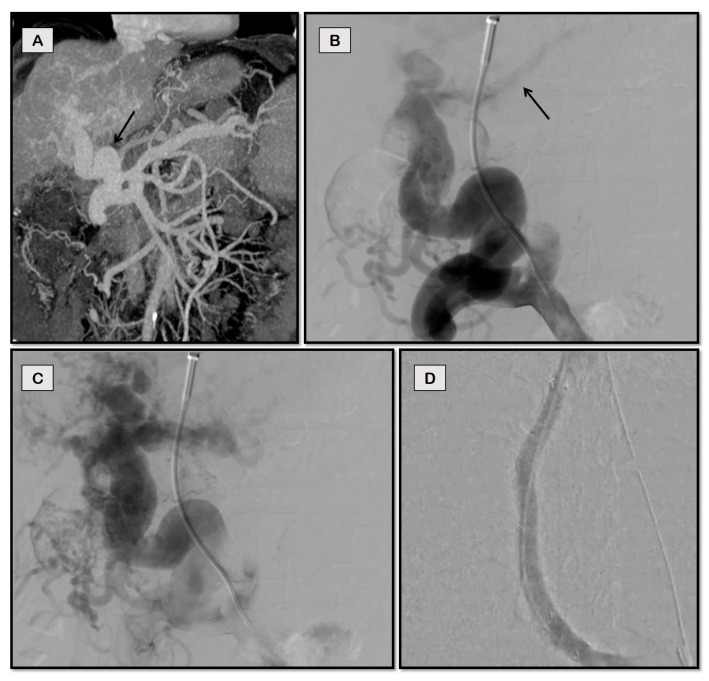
TIPS for refractory ascites in a 53-year-old man with cirrhosis and chronic portal vein thrombosis due to non-alcoholic steatohepatitis (NASH). Coronal-oblique CT image (A) shows a large periportal collateral (arrow) replacing the native main portal vein. The main portal vein was accessed through middle hepatic vein via partially patent left portal vein (faintly opacified on B, arrow) from the jugular approach. Image (C) shows the portal cavernoma. Optimal flow of contrast was noted post-stenting (D) with disappearance of collaterals.
